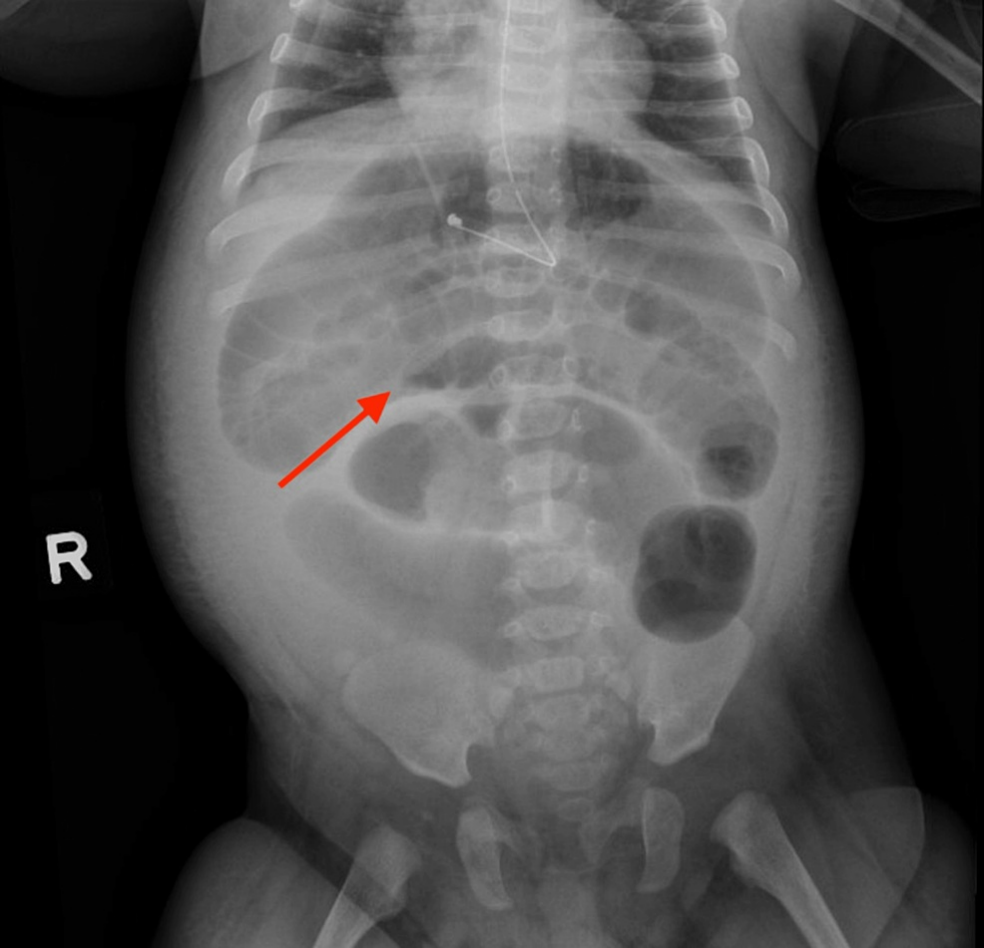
Abdominal radiograph showing marked dilated bowel loops (arrow)
